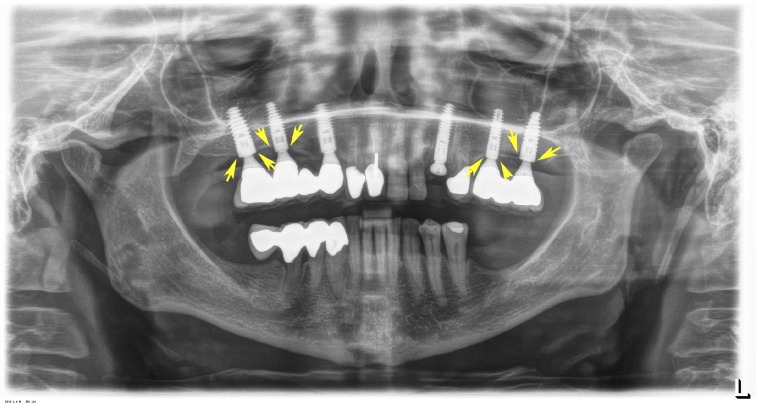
Representative panoramic radiograph showing dental implants without any marginal periimplant bone loss (yellow arrows) 6 years after implant placement in the grafted sinus floors (TCP-P, left; TCP-G, right).
